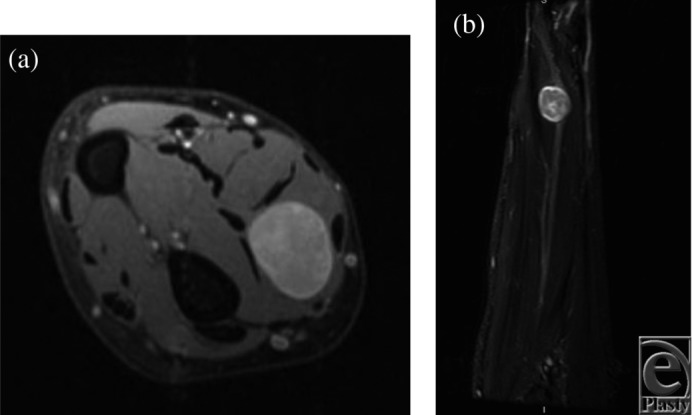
Axial T2 (a) and coronal STIR (b) MRI of the right distal forearm showing a well-circumscribed mass with hyperintense signal. The median nerve is seen in the coronal view with displacement of adjacent tissues.
